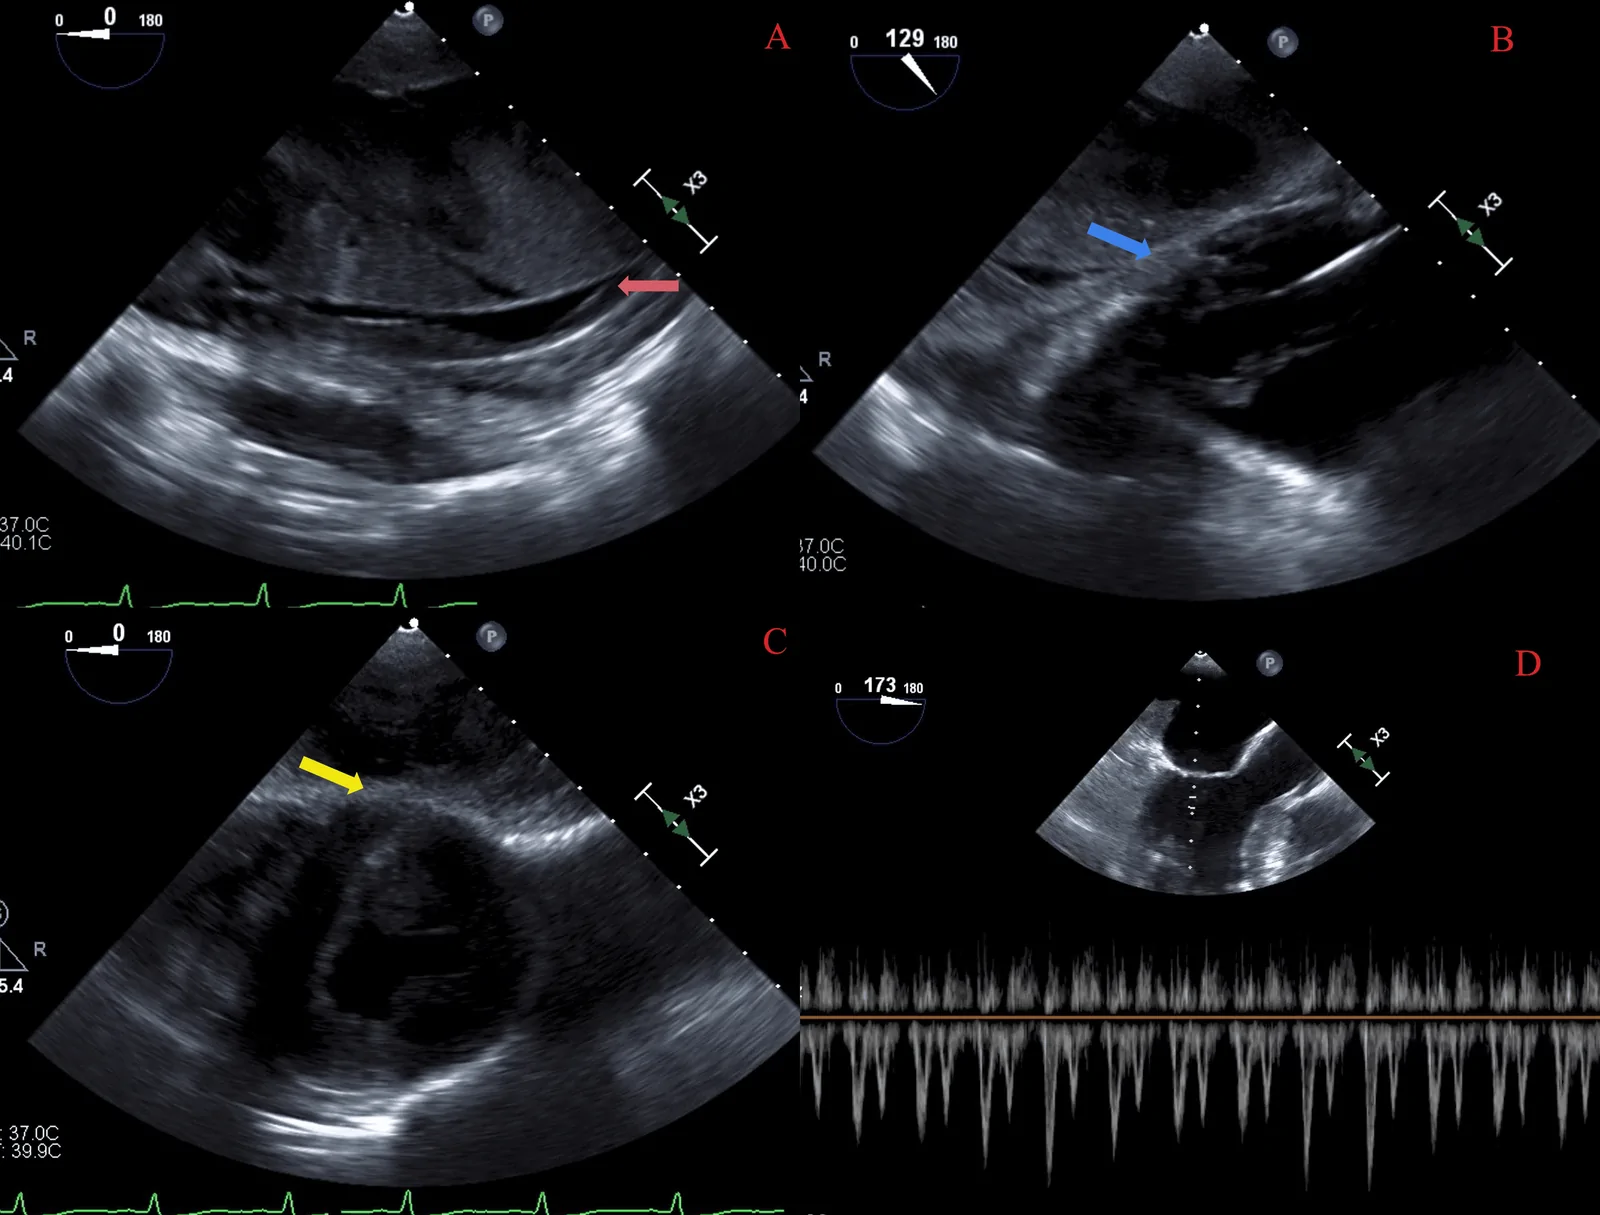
Transesophageal echocardiography. (A) Transgastric view demonstrating hepatic laceration with a subcapsular hematoma (red arrow) causing external compression of adjacent cardiac structures. (B) Transgastric RV inflow view showing extrinsic compression of the RV by the liver (blue arrow). (C) Transgastric mid-papillary short-axis view demonstrating the liver abutting the inferior borders of the RV and LV (yellow arrow), with associated interventricular septal flattening during diastole. (D) Pulsed-wave Doppler of mitral inflow demonstrating significant respiratory variation in E-wave velocity (43%), consistent with tamponade physiology. RV: Right Ventricle; LV: Left Ventricle; E-wave: Early Rapid Filling Wave
